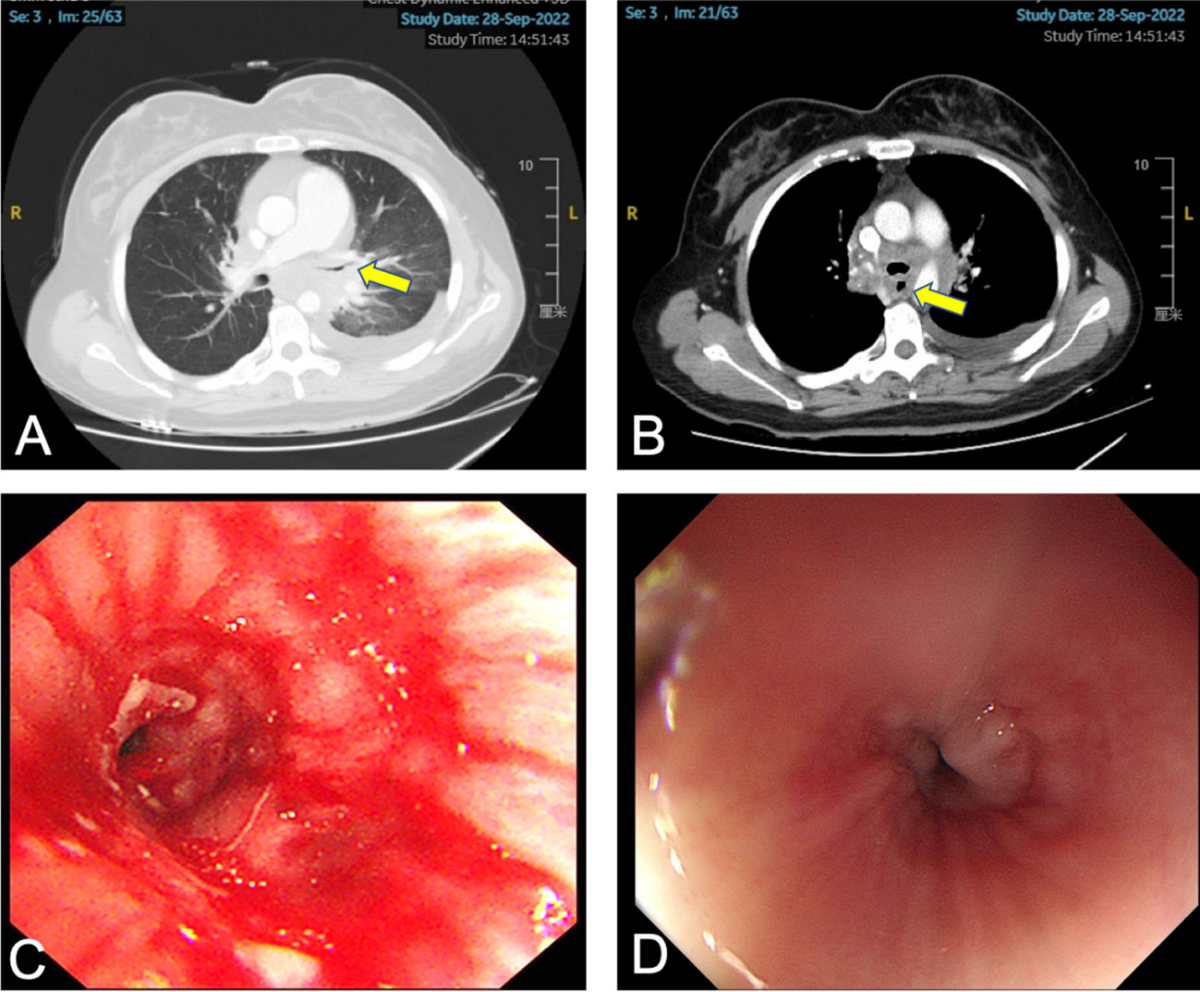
Patient’s chest CT, bronchoscopy, and gastroscopy examination. (A) The arrow indicates the narrowed left main bronchus. (B) The arrow indicates the narrowed esophagus. (C) Bronchoscopy shows narrowing of the left main bronchus with white necrotic material attached. (D) Gastroscopy shows circumferential narrowing of the esophagus with smooth mucosa.
